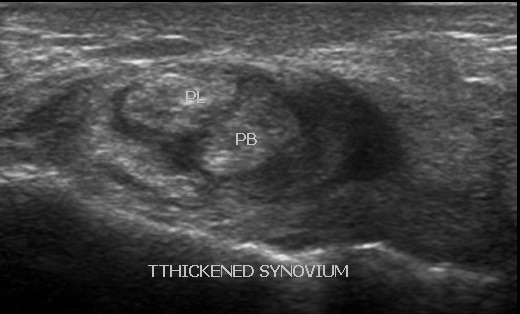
synovial hypertrophy 59 year old female patient with rheumatoid arthritis presented with ankle pain and swelling. Transverse ultrasound of the lateral compartment shows heterogeneously echogenic, non displaceable thickened synovium of the peroneal tendons
